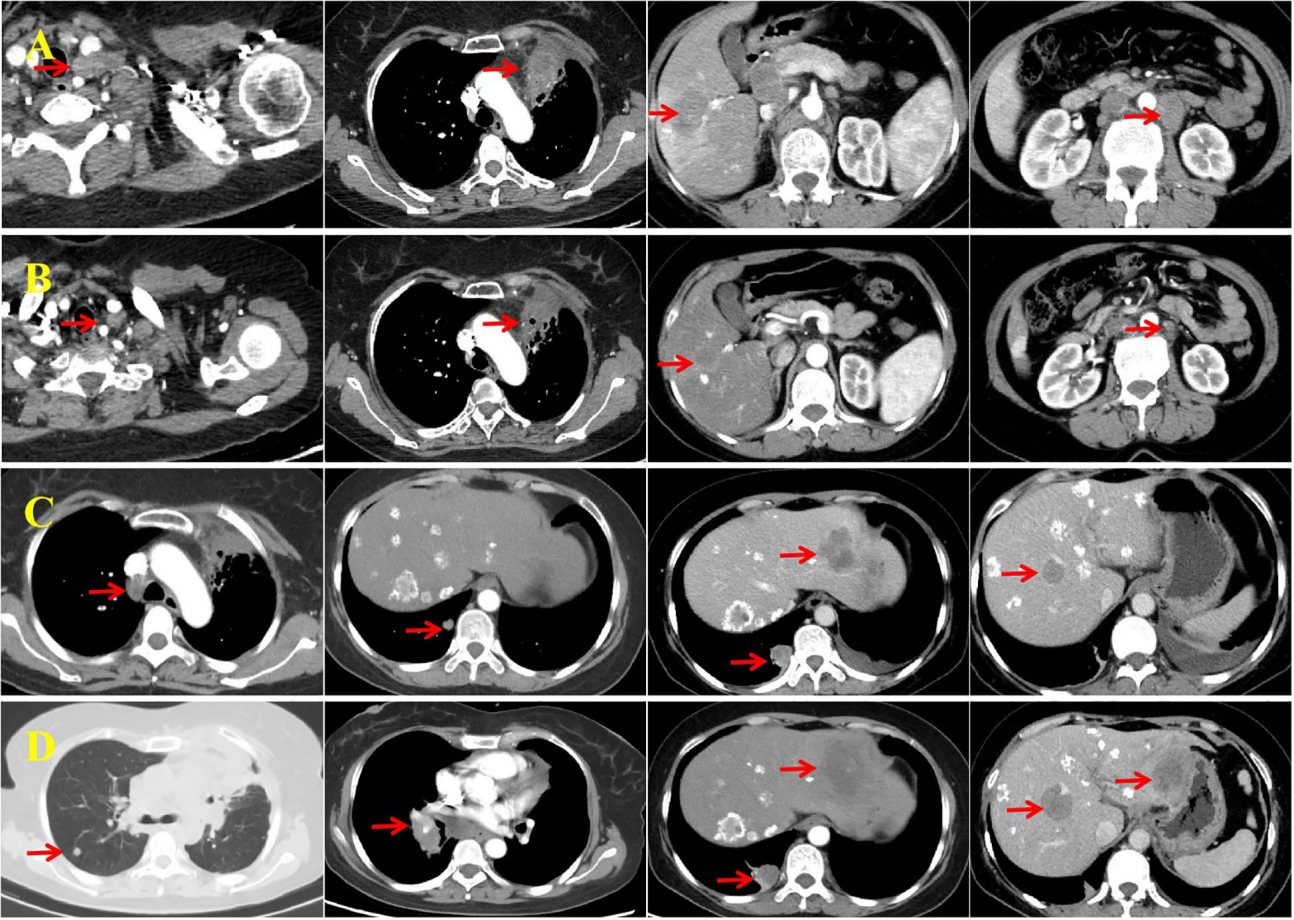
Imaging assessments conducted before and after second- and third-line therapy. (A) Enhanced computed tomography (CT) performed on February 10, 2023 shows progression of the left supraclavicular fossa lymph node and primary lung lesion, along with new liver metastases and enlarged retroperitoneal lymph nodes. (B) After four cycles of second-line therapy, enhanced CT (June 31, 2023) reveals a reduction in the size of all lesions, with the response assessed as a partial response (PR). (C) Enhanced CT on February 14, 2024 shows enlarged mediastinal lymph nodes and new metastases in the lungs and liver, consistent with progressive disease (PD). (D) The most recent CT scan (August 20, 2024) shows further enlargement of mediastinal lymph nodes, increased size and number of bilateral lung metastases, and progression of liver metastases. The imaging confirms PD.
